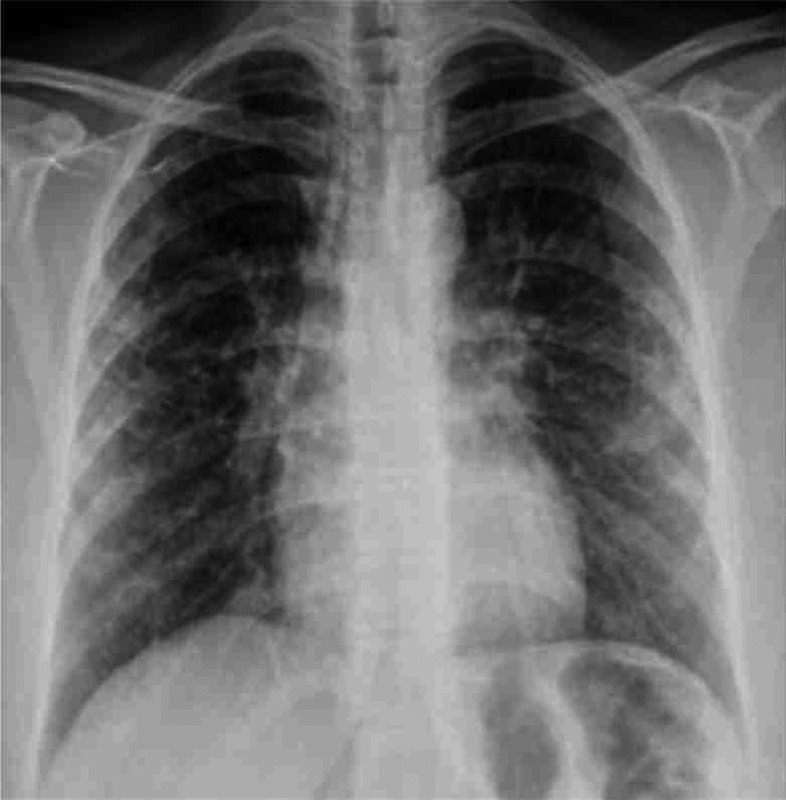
Lung X-ray (after treatment).
